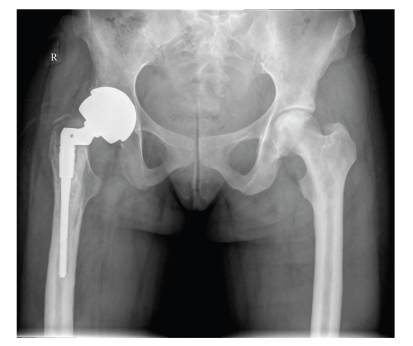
Plain radiograph of the pelvis showing sclerosis of the left femoral head consistent with avascular necrosis. Note total hip replacement due to premature secondary osteoarthritis secondary to avascular necrosis on the right.
